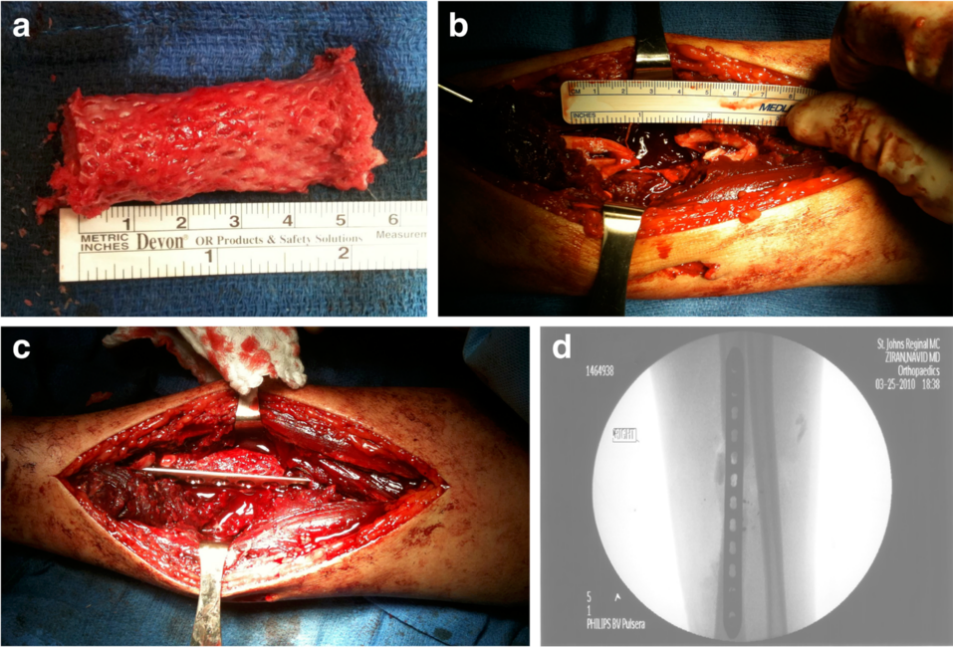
Intra-operative photographs and radiograph intercalary fascial autograft of the right radial shaft. Fascial autograft encasing cancellous autograft and allograft (a). Intra-operative photo demonstrating the size of the right radius bony defect before (b) and after (c) intercalary graft placement and fixation. Intra-operative AP fluoroscopic view of the right forearm (d).
